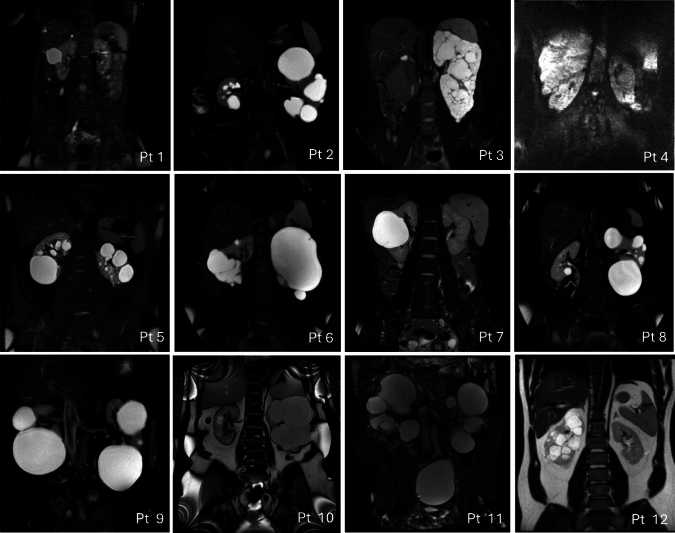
MRI scans of each patient (Pt1-Pt12). (Pt1) Class 2B, bilateral atrophy presentation; (Pt2) Class 2A, lopsided presentation; (Pt3) Class 2A, unilateral presentation; (Pt4) Class 2B, bilateral atrophy presentation; (Pt5) Class 2A, lopsided presentation; (Pt6) Class 2A, lopsided presentation; (Pt7) Class 2A, mild lopsided presentation; (Pt8) Class 2A, asymmetric presentation; (Pt9) Class 2A, lopsided presentation; (Pt10) Class 2A, asymmetric presentation; (Pt11) Class 2A, lopsided presentation; (Pt12) Class 2A, unilateral presentation
